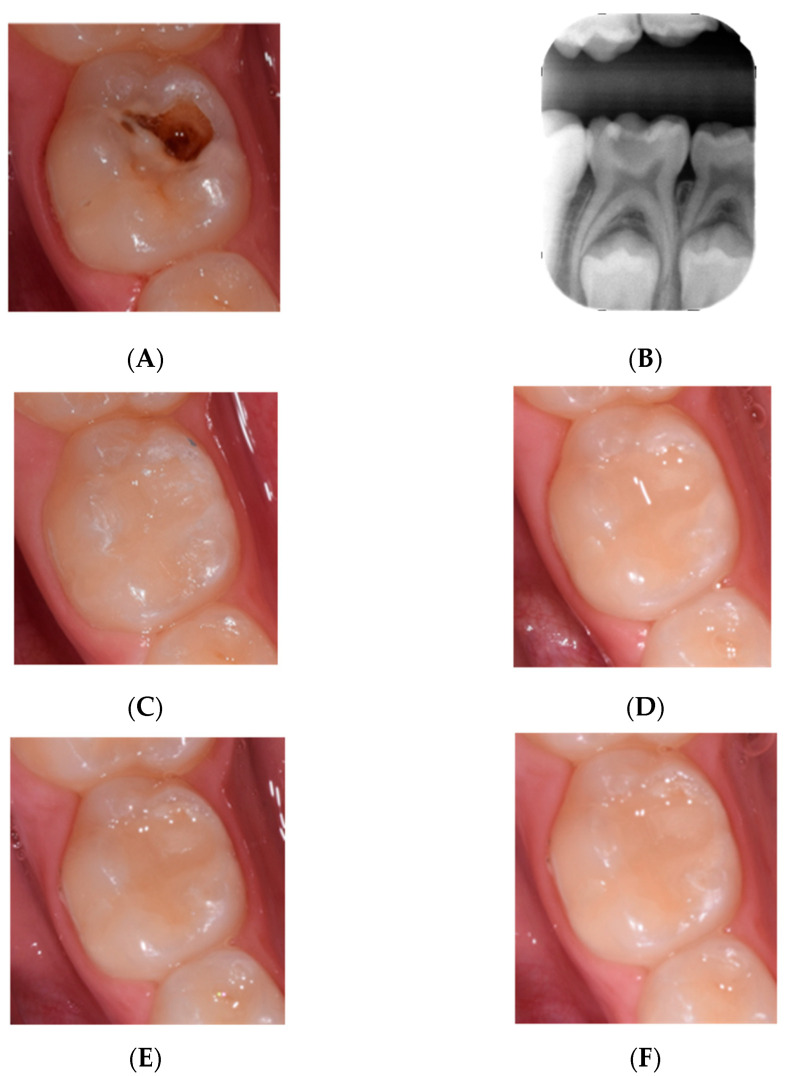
Example of a clinical case in which Activa BioActive was used. (A) pre operatory photograph, (B) pre operatory radiography, (C) post operatory, (D) 3 months post operatory, (E) 6 months post operatory and (F) 1 year follow up.
